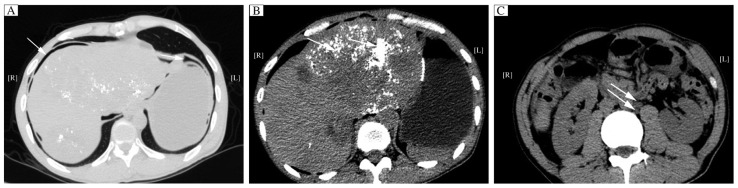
Abdominal pre-contrast CT Scans (PCT). PCT shows little free gas in the subphrenic spaces and around the gastric remnant (A, arrow), multiple liver metastases with inhomogeneous iodized oil drop (B, arrow) and multiple enlarged lymph nodes near the abdominal aorta (C, arrow).
